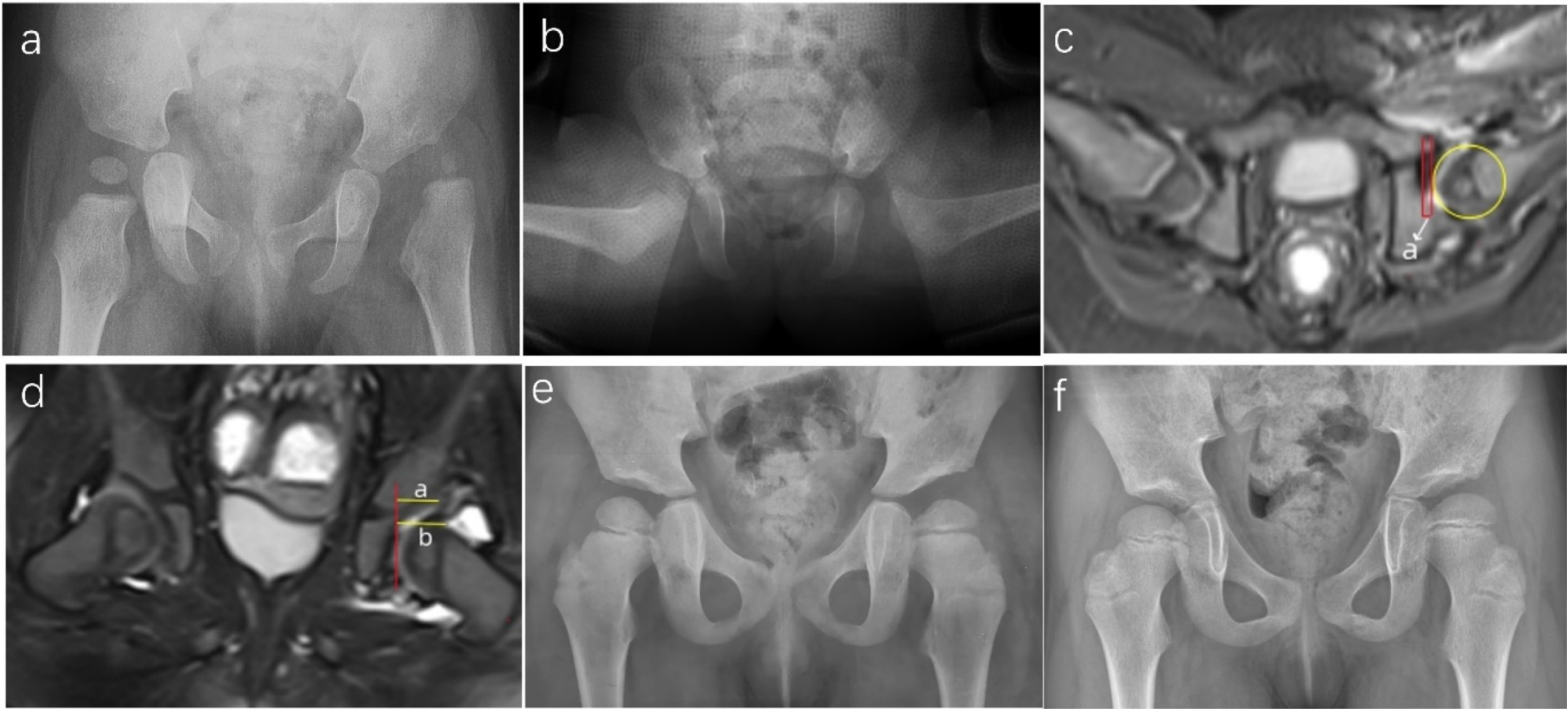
Representative case of successful closed reduction in a girl with left-sided DDH. (a) Preoperative radiograph of a 13-month-old girl showing left-sided DDH. (b) Immediate post-reduction radiograph following spica cast application. (c) Axial postoperative MRI demonstrating concentric reduction of the left hip, with an aAFD of 3.5 mm. (d) Coronal postoperative MRI showing a CAHI of 85% (calculated as a/b × 100). (e) Radiograph at 4 years of age (3 years post-reduction) showing normalization of the femoral head–acetabulum relationship. (f) Radiograph at 6 years and 7 months (5.5 years post-reduction), demonstrating a Severin Grade I outcome for the left hip.
